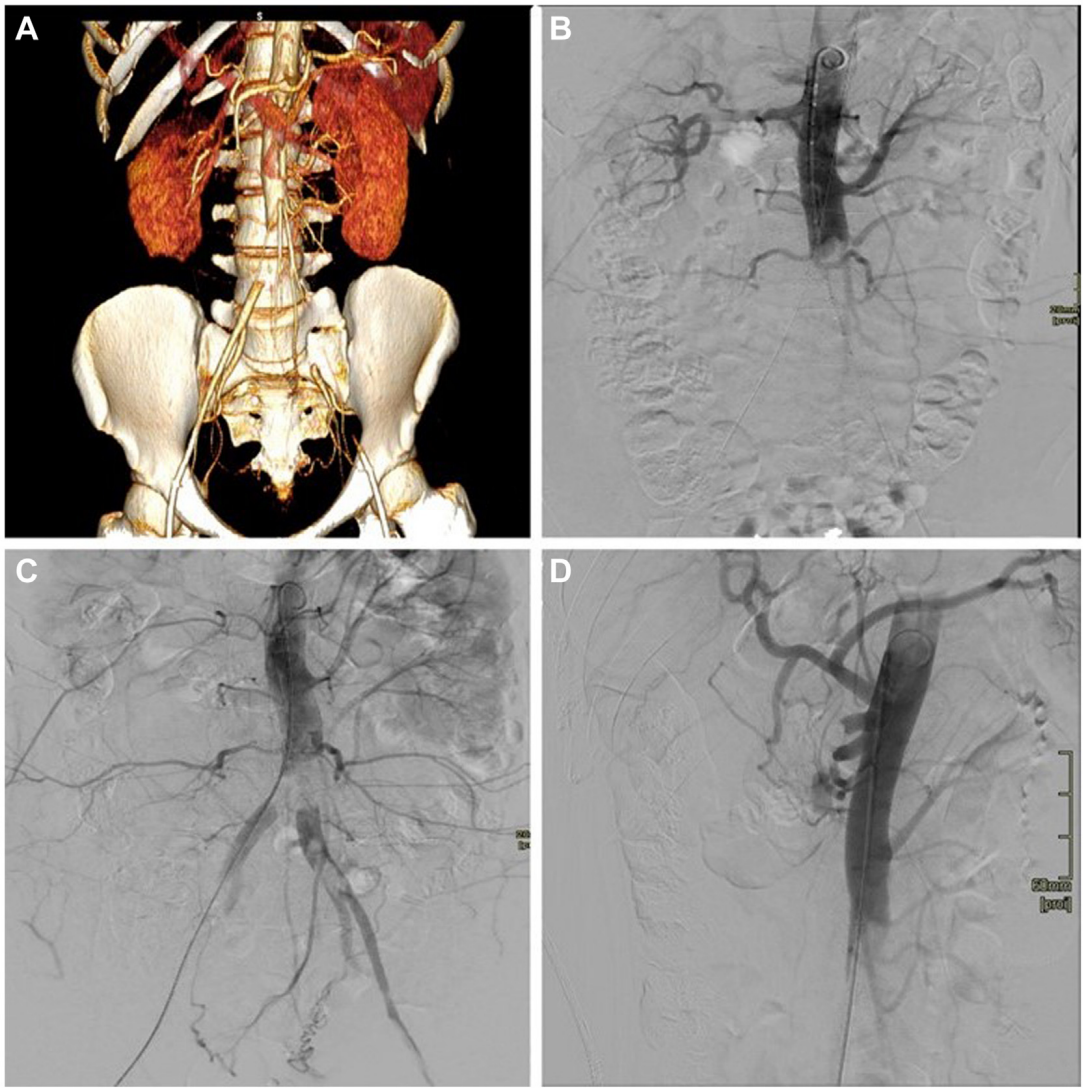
A, Three-dimensional reconstruction of computed tomography angiogram at first emergency department visit. B, Angiogram showing visceral and aortoiliac thrombosis. C,D, Final angiogram showing residual renal, mesenteric, and iliac thrombosis.
